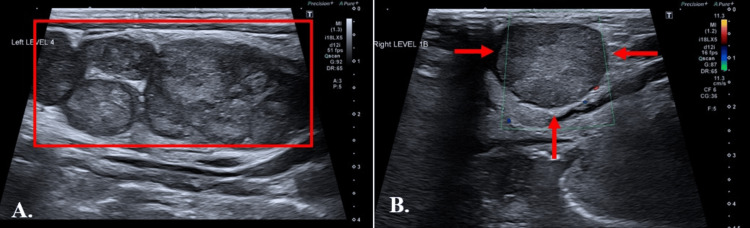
Ultrasound of the neck showing (A) multiple enlarged, heterogeneous cervical lymph nodes arranged in a matted cluster (boxed) and (B) an enlarged right submandibular lymph node (arrows).
